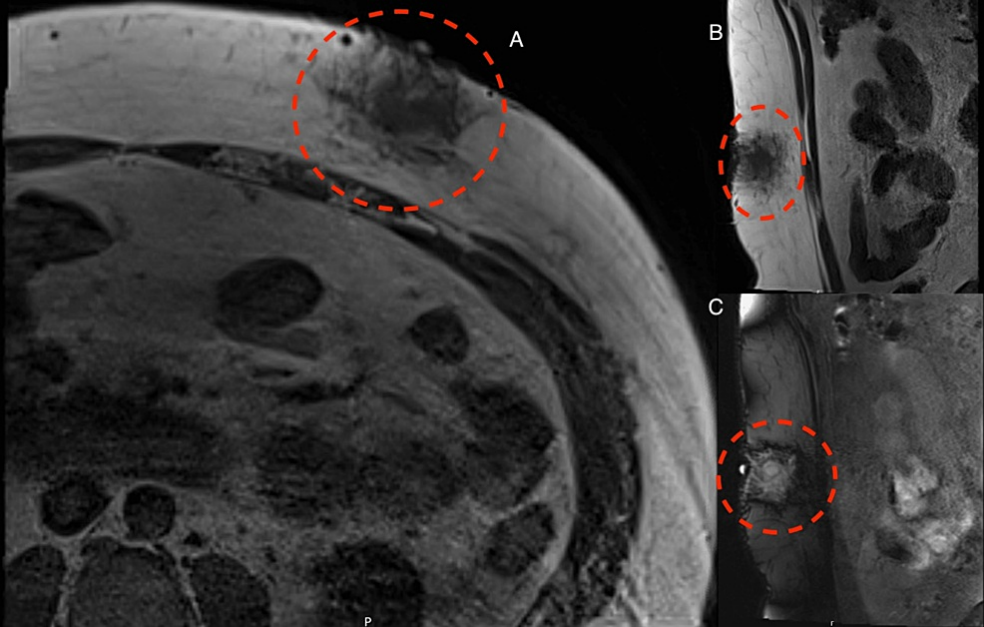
T1- and T2-weighted MRI of abdomen Irregular hyperintense lesions on cross-sectional T1 (A) and sagittal T1 (B). Sagittal T2 (C) with lesion heterogeneity noted The mass is seen involving the left lower anterior abdominal wall with no detectable hemorrhagic components
